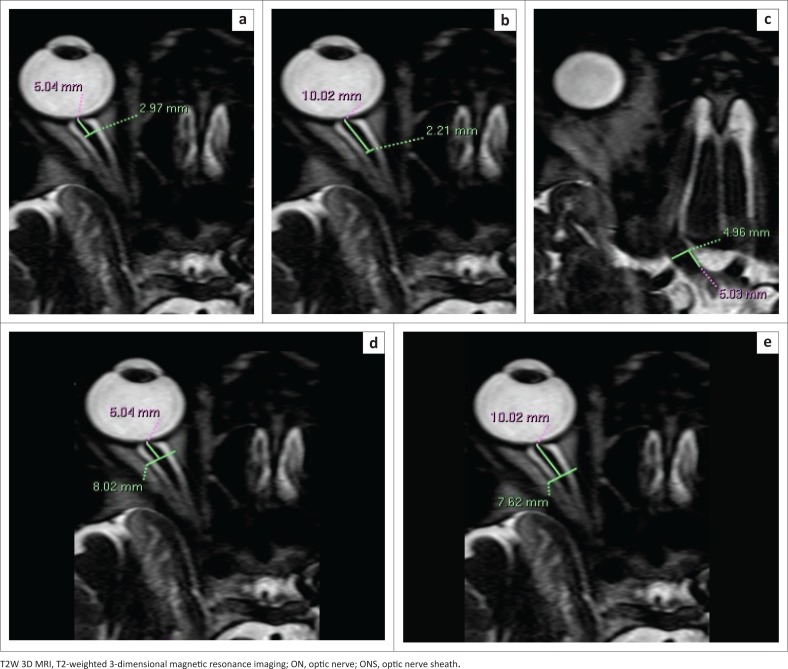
Axial T2W 3D magnetic resonance imaging of the right orbit demonstrating the measurement technique: (a and b) intraorbital optic nerve width 5 mm and 10 mm behind the globe, respectively; (c) intracranial optic nerve width and (d and e) optic nerve sheath width 5 mm and 10 mm behind the globe, respectively.
